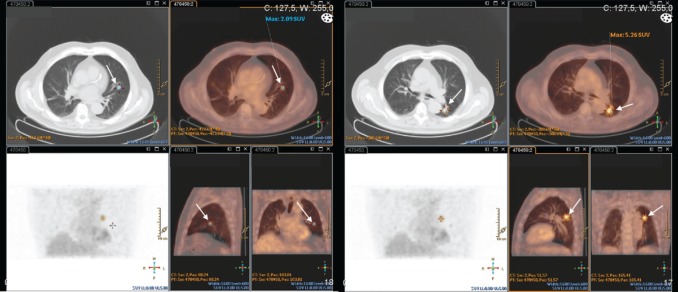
Patient #3 PET-CT-FDG: two hypermetabolic nodes in the lower lobe of the left lung – apical and anterobasal segments (arrows).
